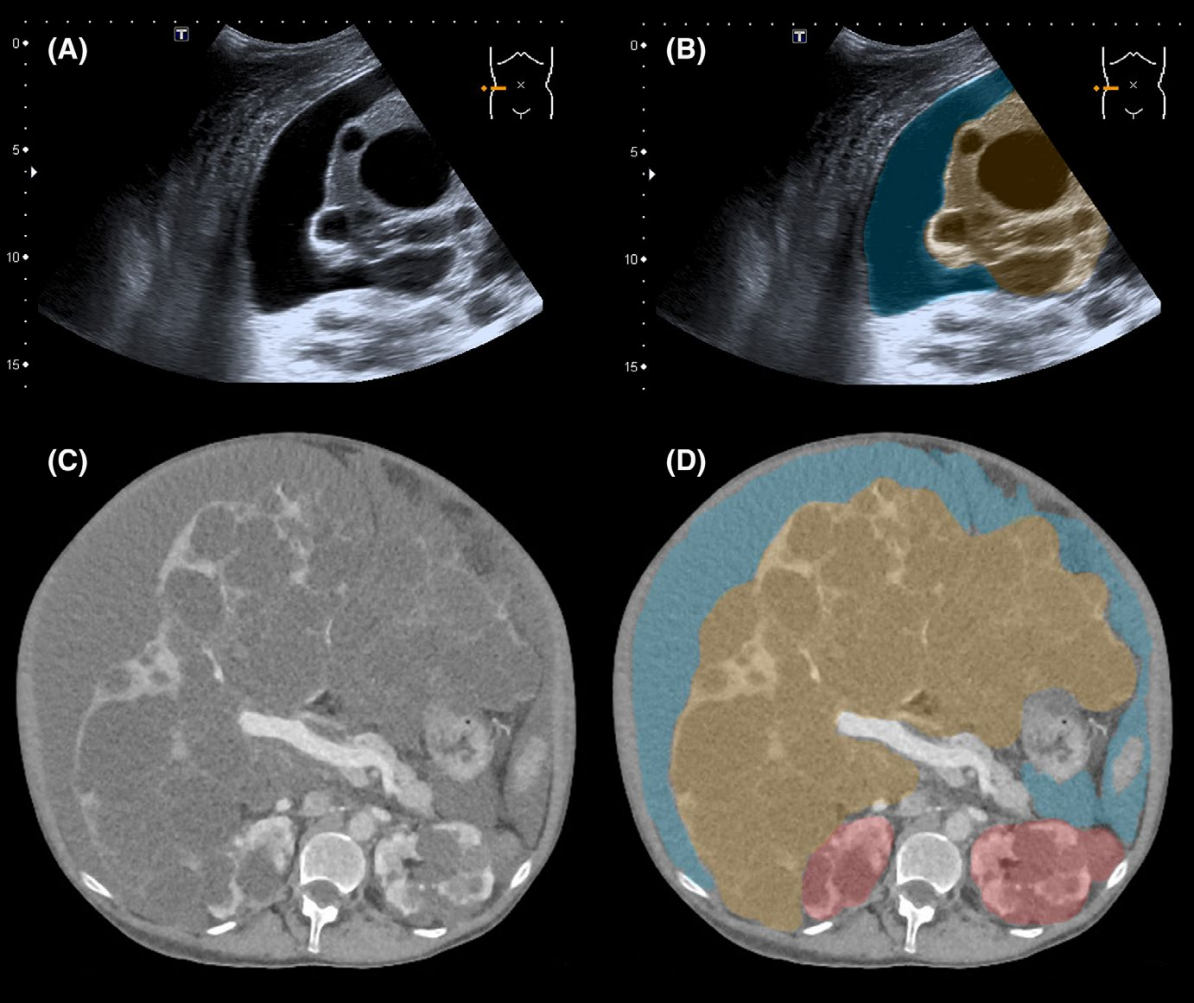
Imaging with ultrasound and transversal computed tomography (CT) of ascites in a polycystic liver disease patient with hepatic venous outflow obstruction. Ascites is coloured blue, polycystic liver tissue is coloured orange, polycystic kidney tissue is coloured red
